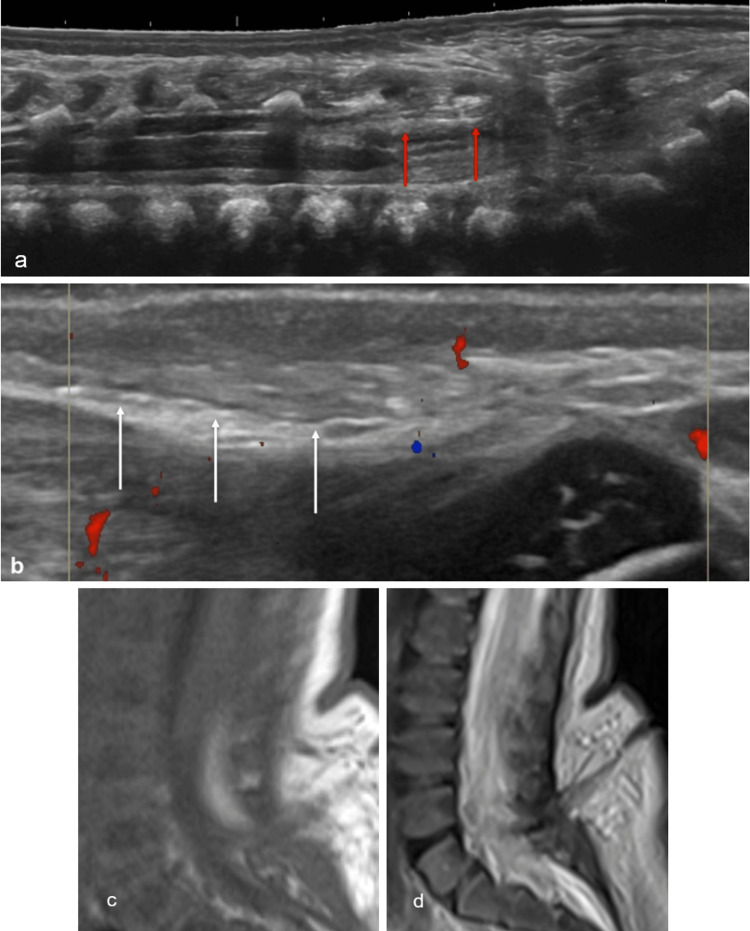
Dorsal dermal sinus Longitudinal (a, b) ultrasound images demonstrate a low-lying conus with an echogenic structure dorsally within the spinal canal below the level of the conus (red arrow). Also noted is a linear tract extending into the subcutaneous plane (white arrow). Sagittal T1 (c) and T2 (d) MR images confirm the low-lying conus at L4 and the hypoechoic tract connecting the skin to the distal thecal tissues at L5-S1, at the site of bulging of soft tissues, compatible with the patient’s sacral dimple Radiological images obtained by the authors
